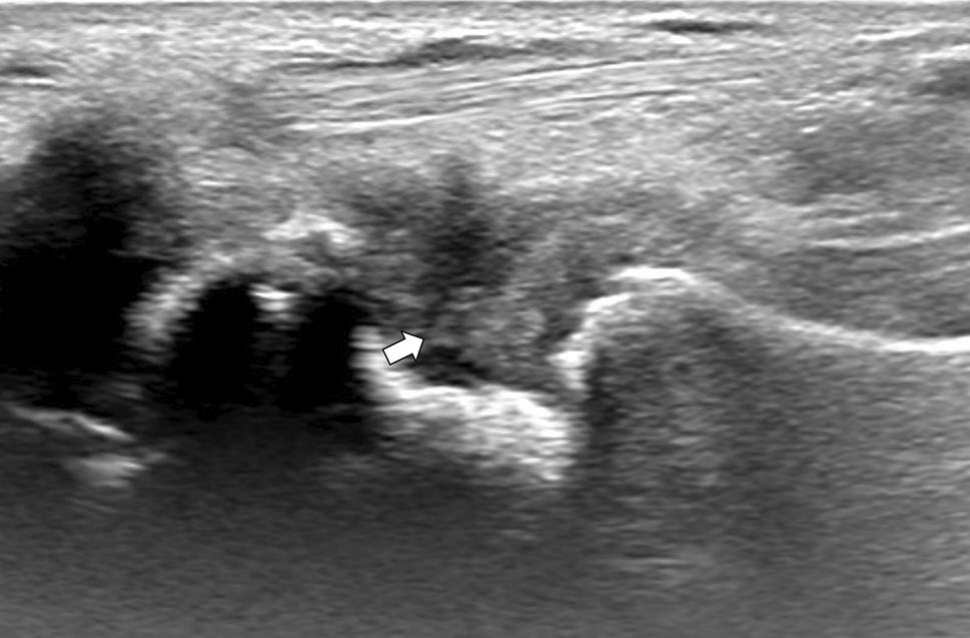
Synovial hypertrophy and joint effusion revealed in ultrasonography of the radiocarpal joint and intercarpal joint on the palmar side
